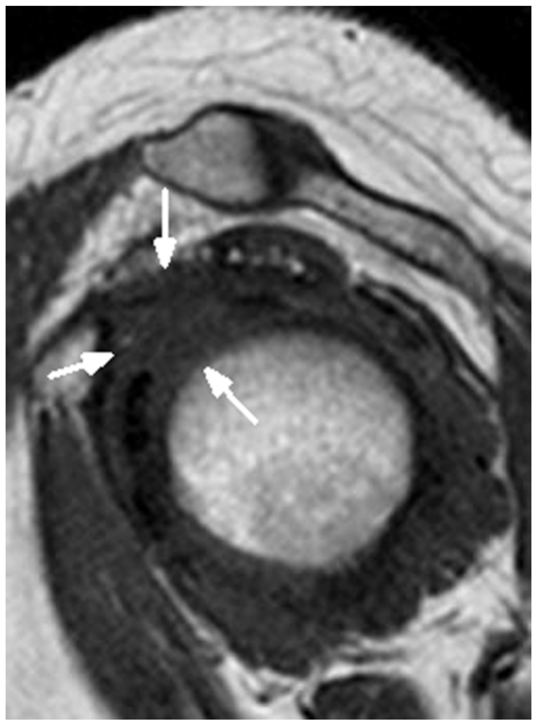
A 57-year-old male patient with clinical evidence of right frozen shoulder. Sagittal oblique, T1-weighted image (TR/TE = 550 ms/15 ms) shows the complete obliteration of subcoracoid fat triangle and distinct fatty tissue surrounding the CHL as having disappeared, and the CHL cannot be measured (arrows).
